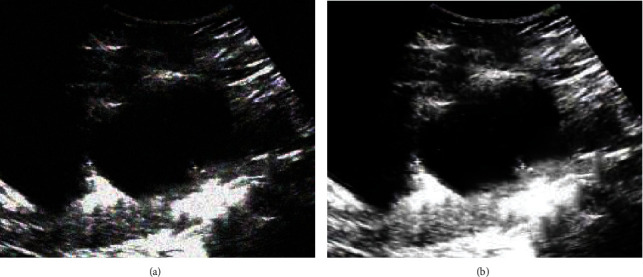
Comparison of ultrasound imaging results before and after optimization of the improved UNet algorithm. (a) was the original ultrasound image; (b) was the ultrasound image denoised by the improved UNet algorithm.
